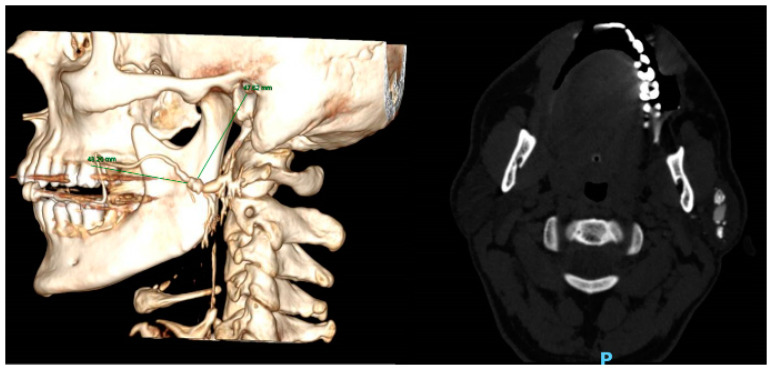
Computed Tomography (CT) Sialography of a left protid gland sialolith. Showing distance of stone from external acoustic meatus (47.62 mm) and from the second molar (43.20 mm).
